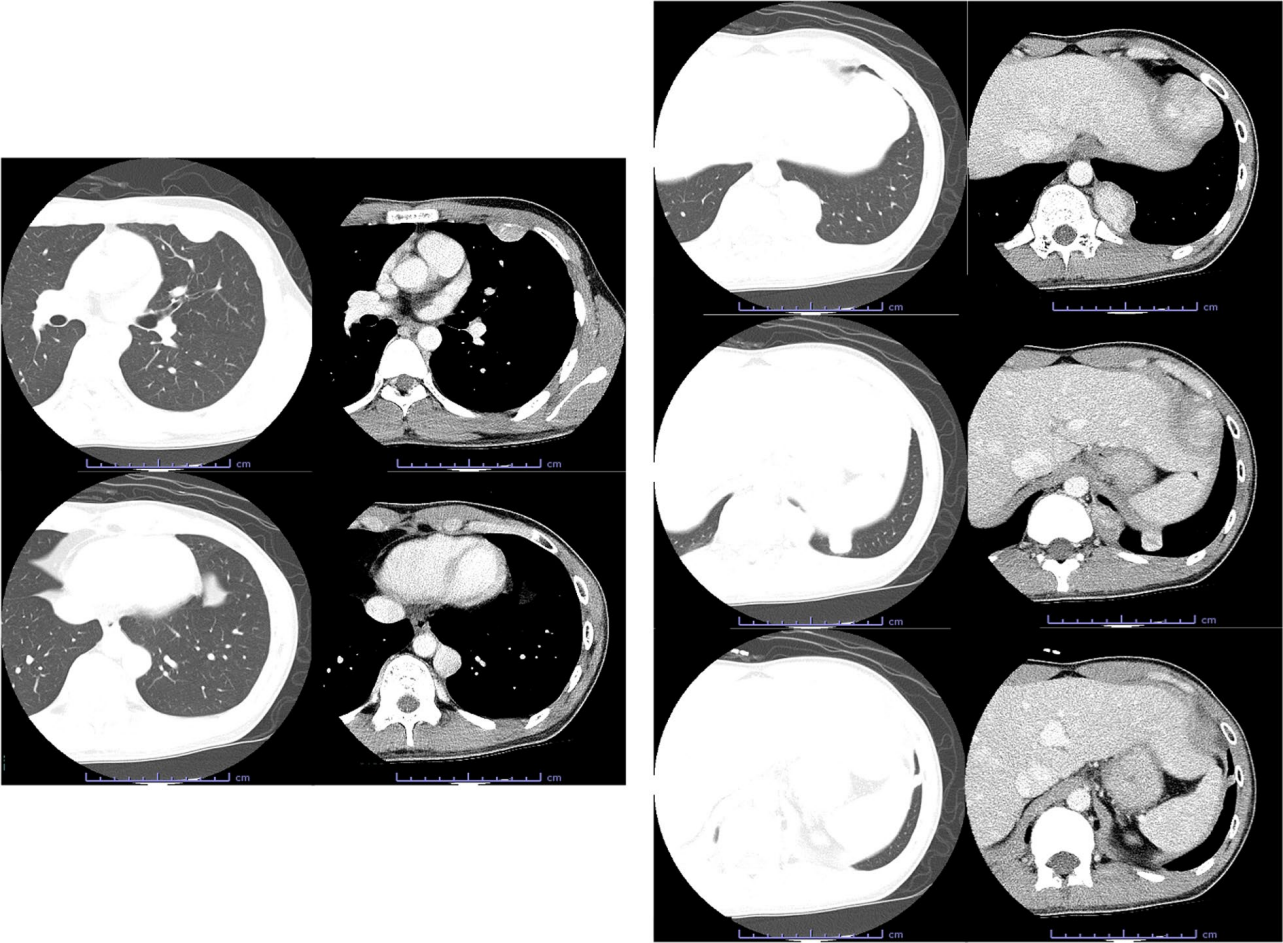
Chest CT showed 6 tumors in the left thoracic cavity. These tumors were well defined, noninvasive, lobular, noncalcified, adjacent to the chest wall, and unevenly enhanced on contrast-enhanced CT
